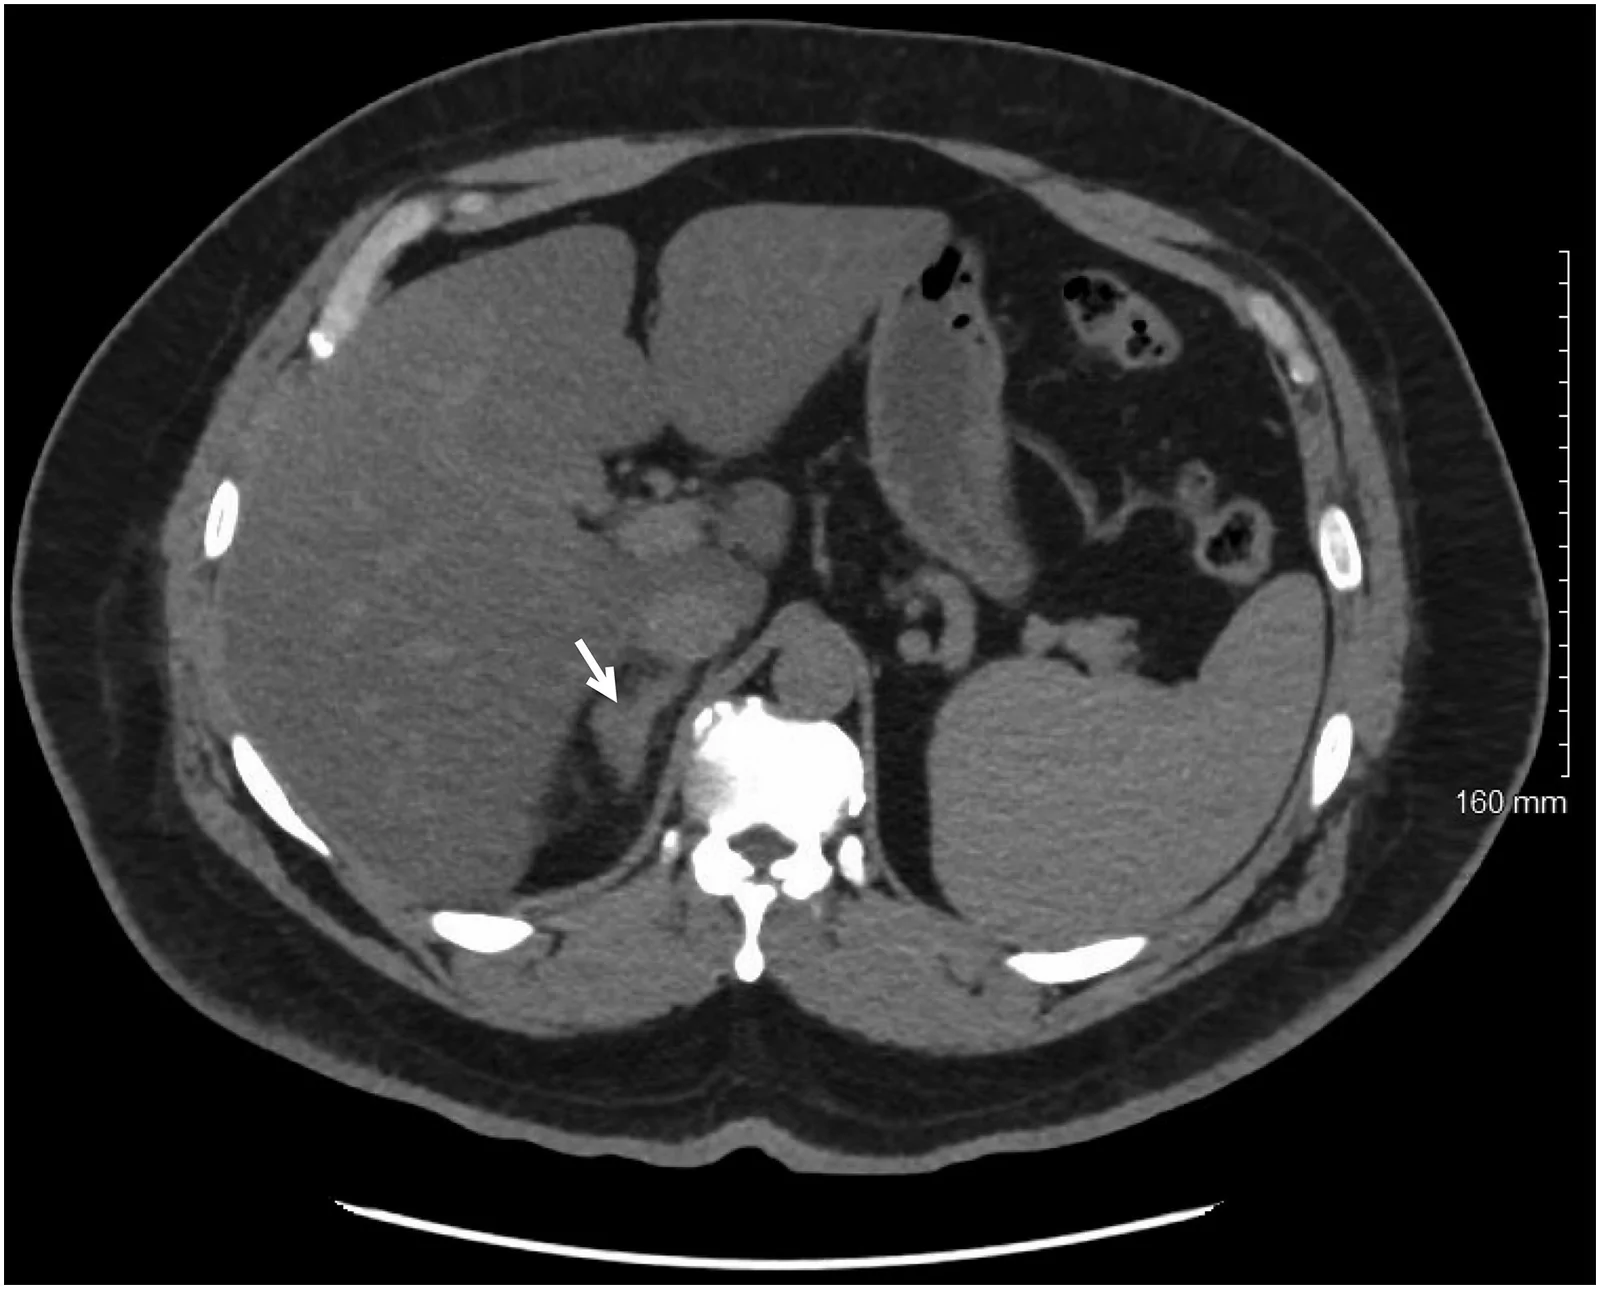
Computed tomography abdomen pelvis without contrast: mild fat stranding surrounding the right adrenal gland, most likely postprocedural. No evidence of significant hematoma or active contrast extravasation.
